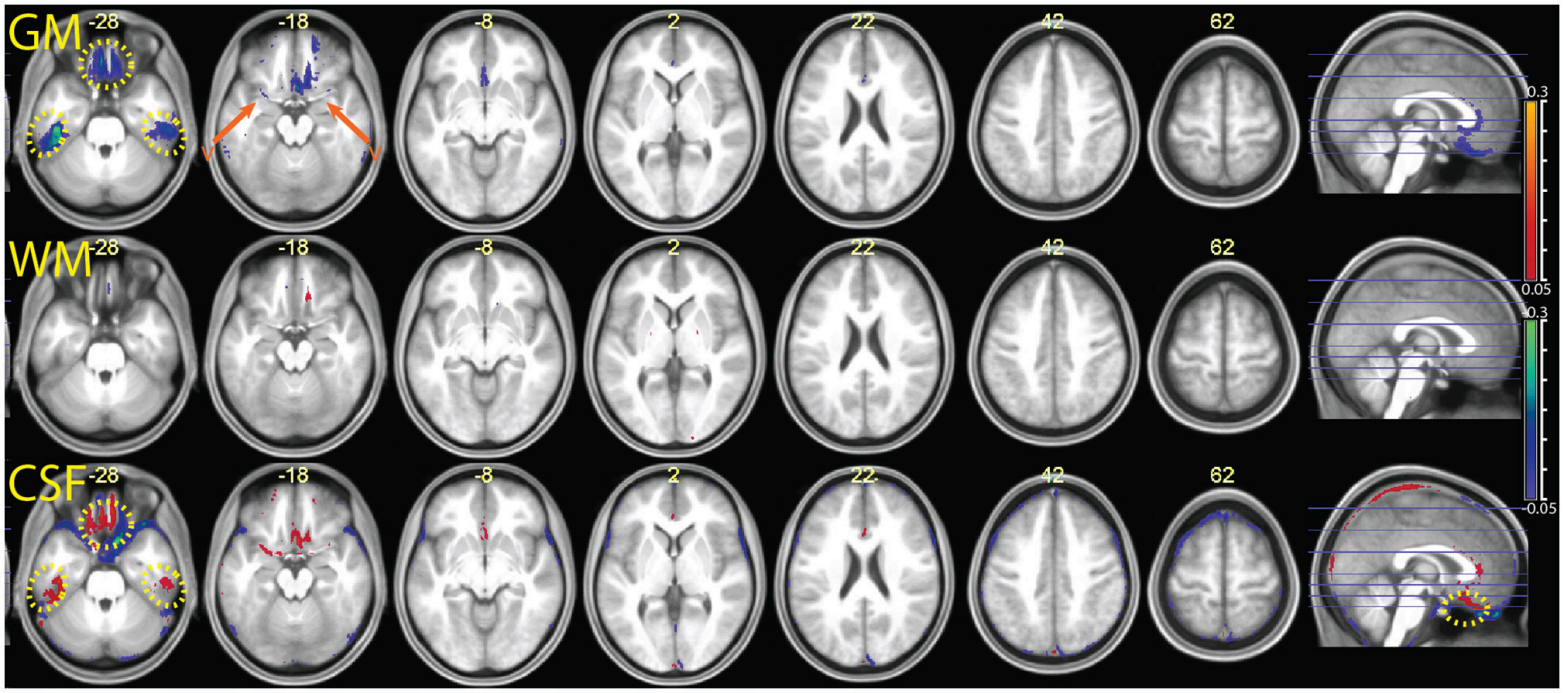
Comparison of the segmentation obtained with the multimodal procedure using MPRAGE images, FLAIR images, and the ${\text{R}}_{2}^{*}$ maps, relative to the segmentation obtained with the combination MPRAGE + FLAIR images. Decreases of estimated GM volume amounted to an average 9 ± 9 ml in the MPRAGE+FLAIR+${\text{R}}_{2}^{*}$ relative to MPRAGE+FLAIR. There were no appreciable differences in the WM volumes in the combined and simple segmentation (<1 ml). Effects on the CSF volume were not consistent. In the MPRAGE+FLAIR+${\text{R}}_{2}^{*}$ segmentation, CSF volumes increased on average by 14 ± 28 ml than in the MPRAGE+FLAIR segmentation. Colors and symbols as in Figure 5.
